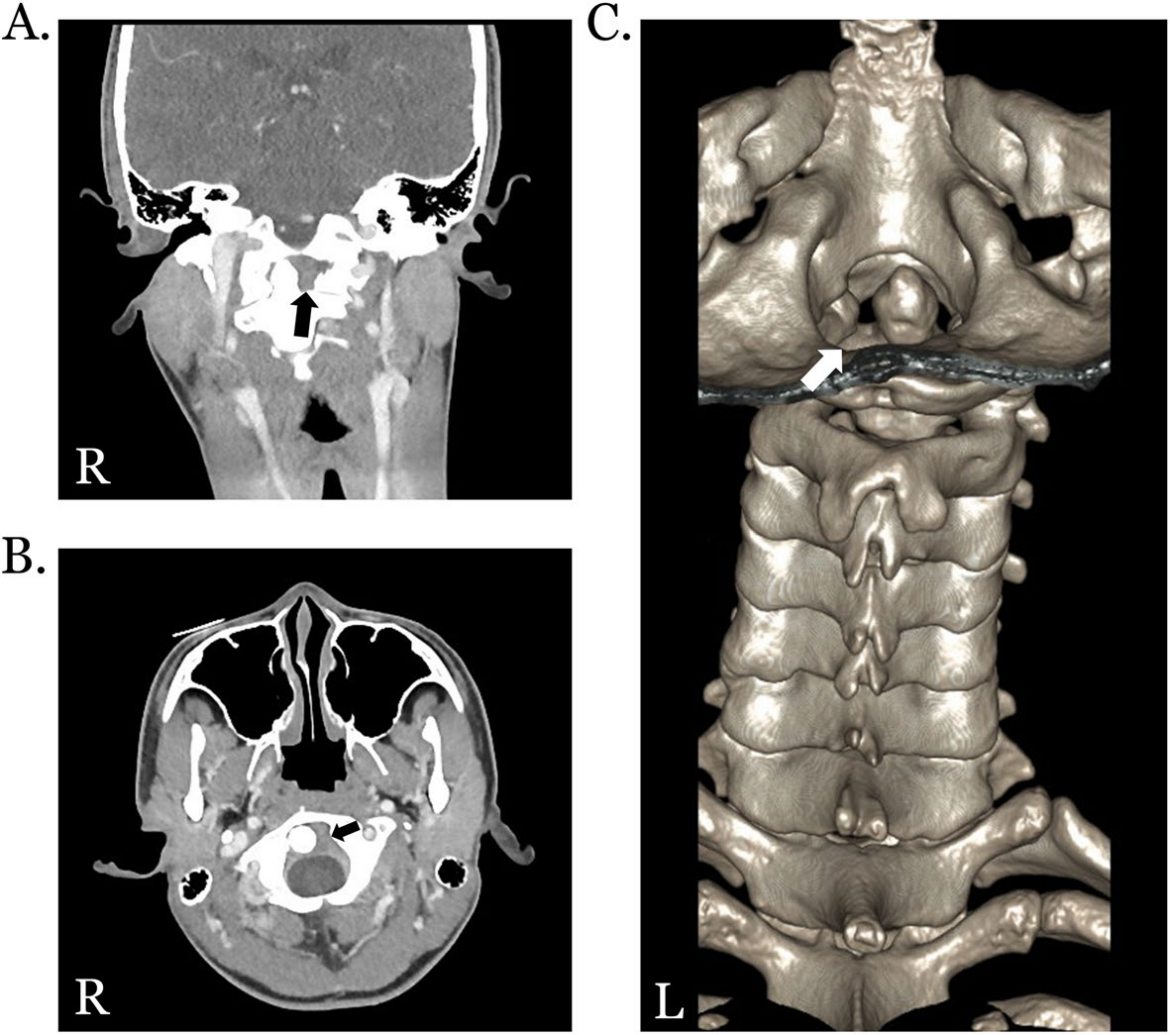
Computed tomography of cervical spine showing C1-C2 subluxation in the present patient with enthesitis-related arthritis with bilateral sacroiliitis. *Computed tomography (CT) of the cervical spine shows widened left interspace between the odontoid process and arches of the atlas in coronal view (black arrow, A) and in axial view (black arrow, B). Three-dimensional reconstruction of cervical spine CT also indicates widened lateral space in the left interspace between the odontoid process and arches of the atlas (white arrow, C). R: Right; L: Left*
